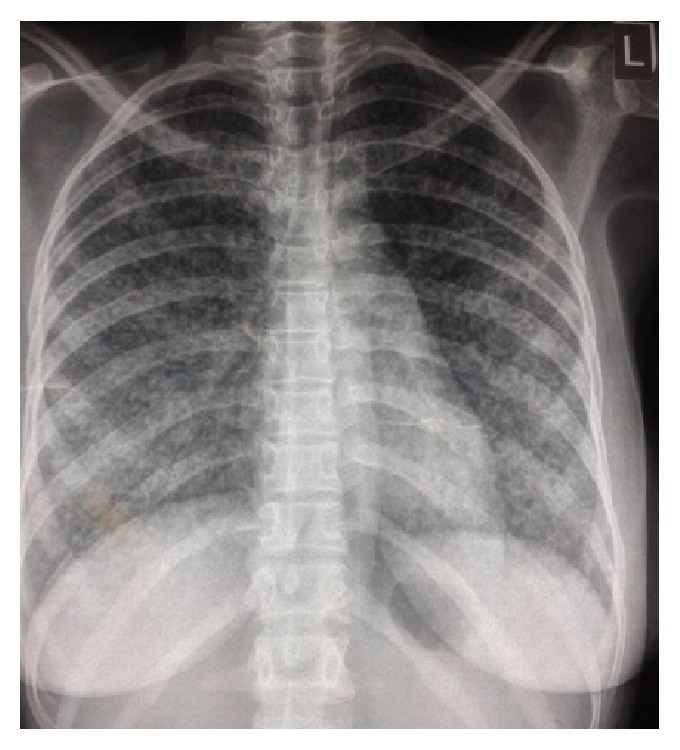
Chest radiograph showing widespread (whole lung) reticuloalveolar “miliary” infiltrates.
